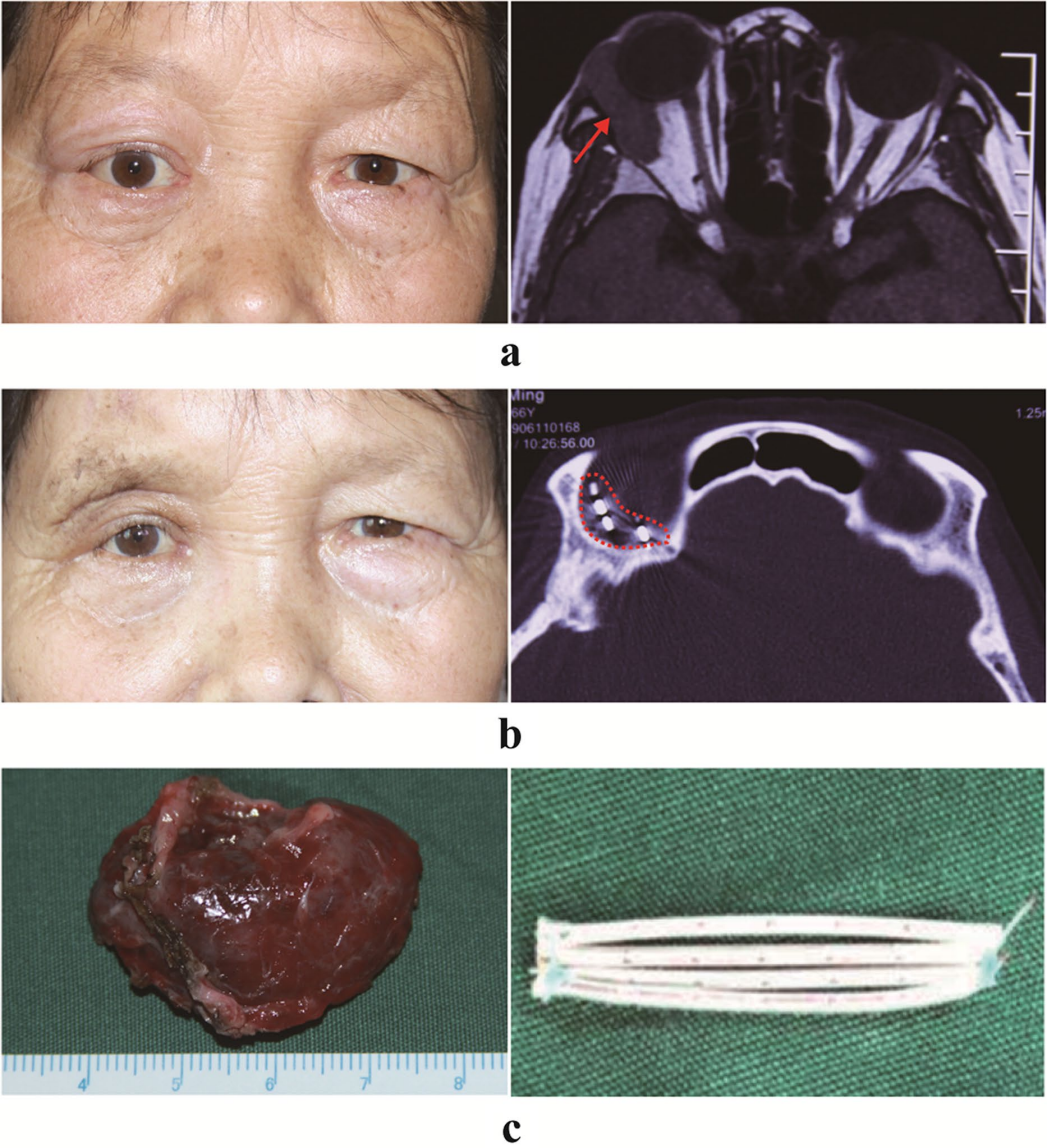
Pre- and postoperative images of patient A. a Preoperative images of patient A. The photograph of the patient's appearance shows the right eyeball protruding with eyelid swelling. A CT scan shows a soft tissue mass in the right orbit (red arrow) and squeezing the right eyeball. b Seventeen months after brachytherapy. The photograph of the patient's appearance shows no proptosis and no periocular radiation dermatitis. The CT scan shows that the I-125 seeds under the orbital periosteum are stable (red dashed box). c The tumor was resected and the size was measured. The seed tube is in preparation
